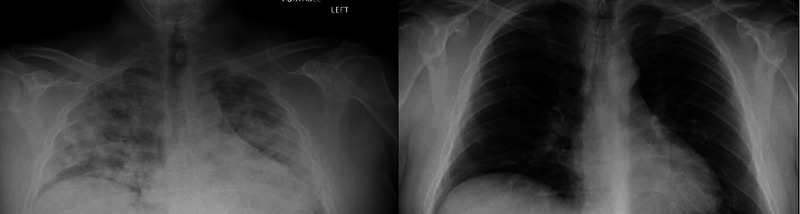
Chest X-ray on the left shows bilateral pneumonia, and chest X-ray on right shows clearance of pneumonia.
